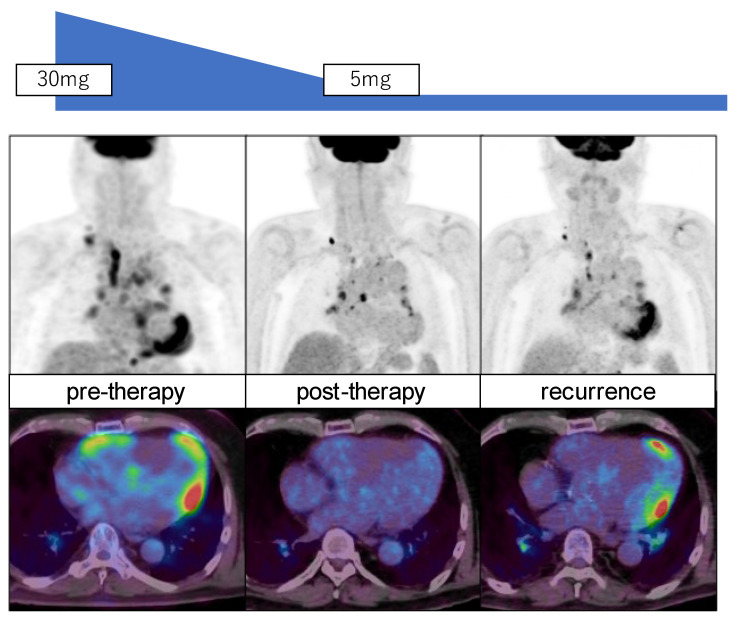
FDG PET MIP images (top) and transaxial images (bottom) of a patient with sarcoidosis before (left) and after steroid (prednisolone) therapy (middle) and in recurrent stage (right). Note significant reduction in FDG uptake in the myocardium and lymph nodes post-therapy with increased uptake in the myocardium with recurrence.
